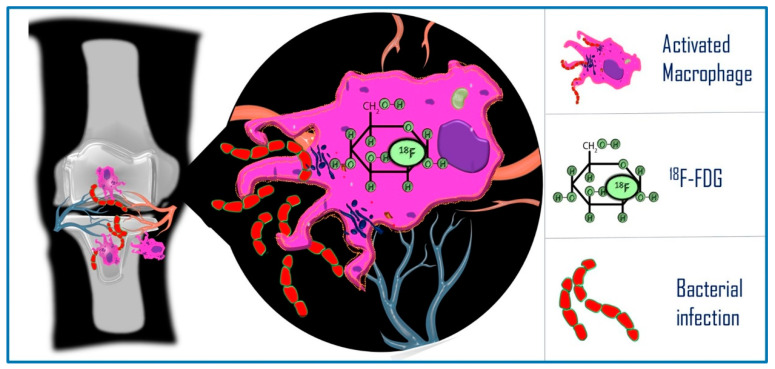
Mechanism of uptake of ^18^F-FDG-labeled white blood cells in infectious foci.
